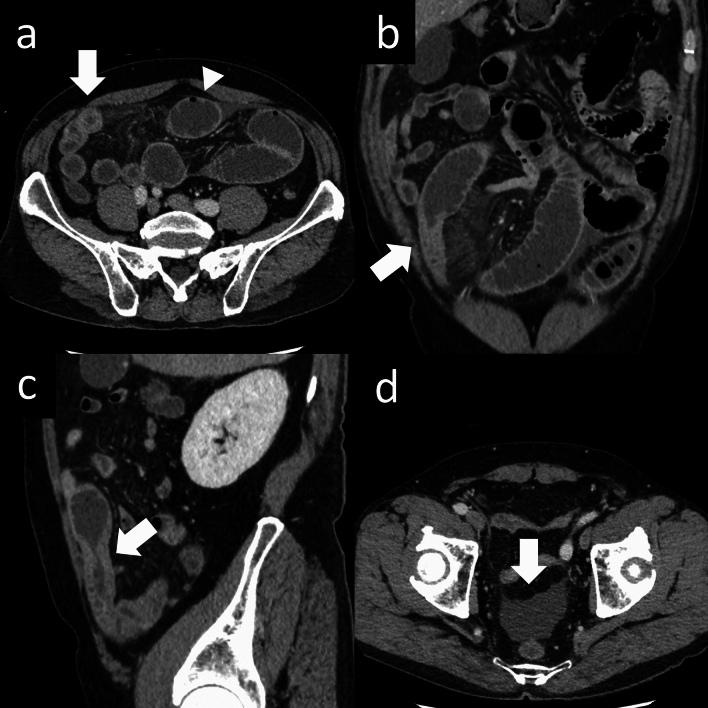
A 56-year-old man presented to the emergency department with a complaint of abdominal pain since the previous day. Axial post-contrast CT images show edematous wall thickening of the small intestine (Fig. 4a, arrow), along with proximal small bowel dilatation (Fig. 4a, arrowhead) and increased fat density. Coronal and sagittal post-contrast CT images demonstrate progressive intestinal wall thickening at the site of caliber change (clamp sign, Fig. 4b, c, arrows). Ascites is noted in the pelvic cavity (Fig. 4d, arrow)
